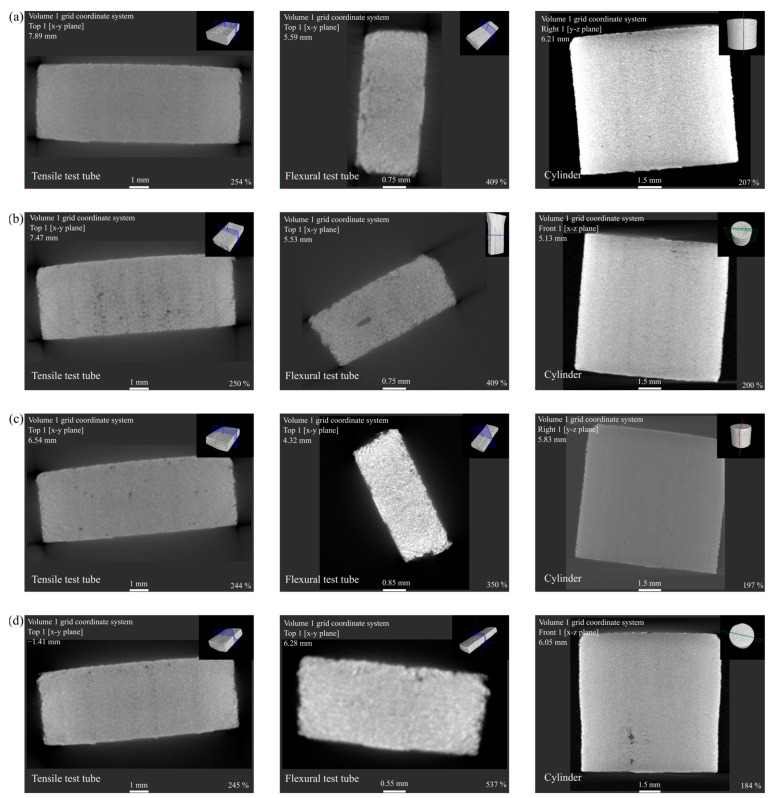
CT scan comparison of FDM-printed samples in batches: (a) MQP S/PA12, (b) MQP S/PA12 CA, (c) MQP S/PA12*, and (d) MQP S*/PA12*.
